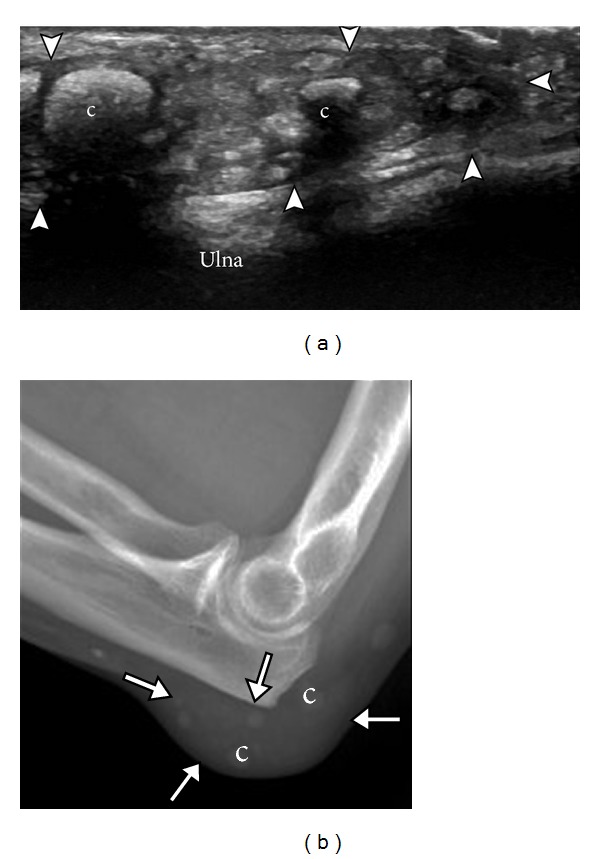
Olecranon bursa. Ultrasound (a) and radiograph (b) demonstrate olecranon bursa (arrowheads, arrows) overlying ulna. Note multiple soft tissue nodules in the bursa, some partially calcified (c).
